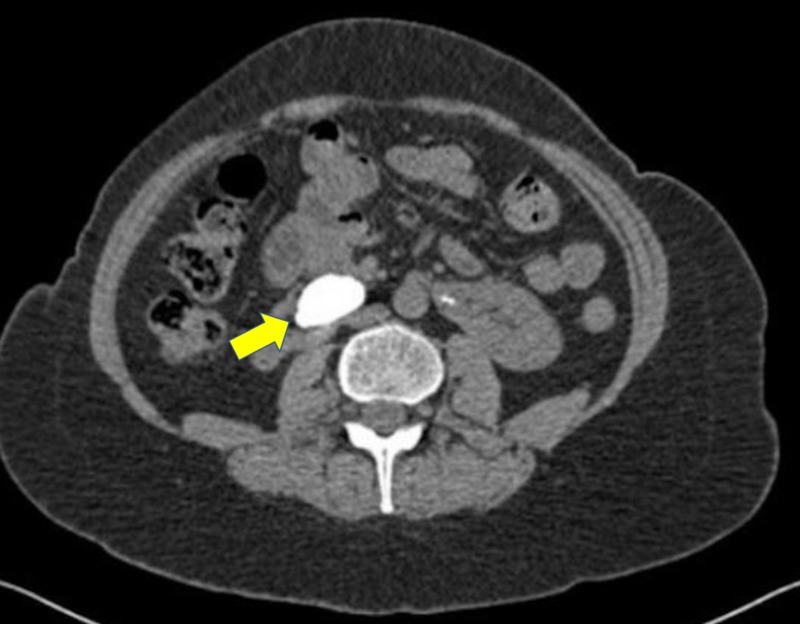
Non-contrast CT scan demonstrating a large calculus in the right HSK HSK, horseshoe kidney
